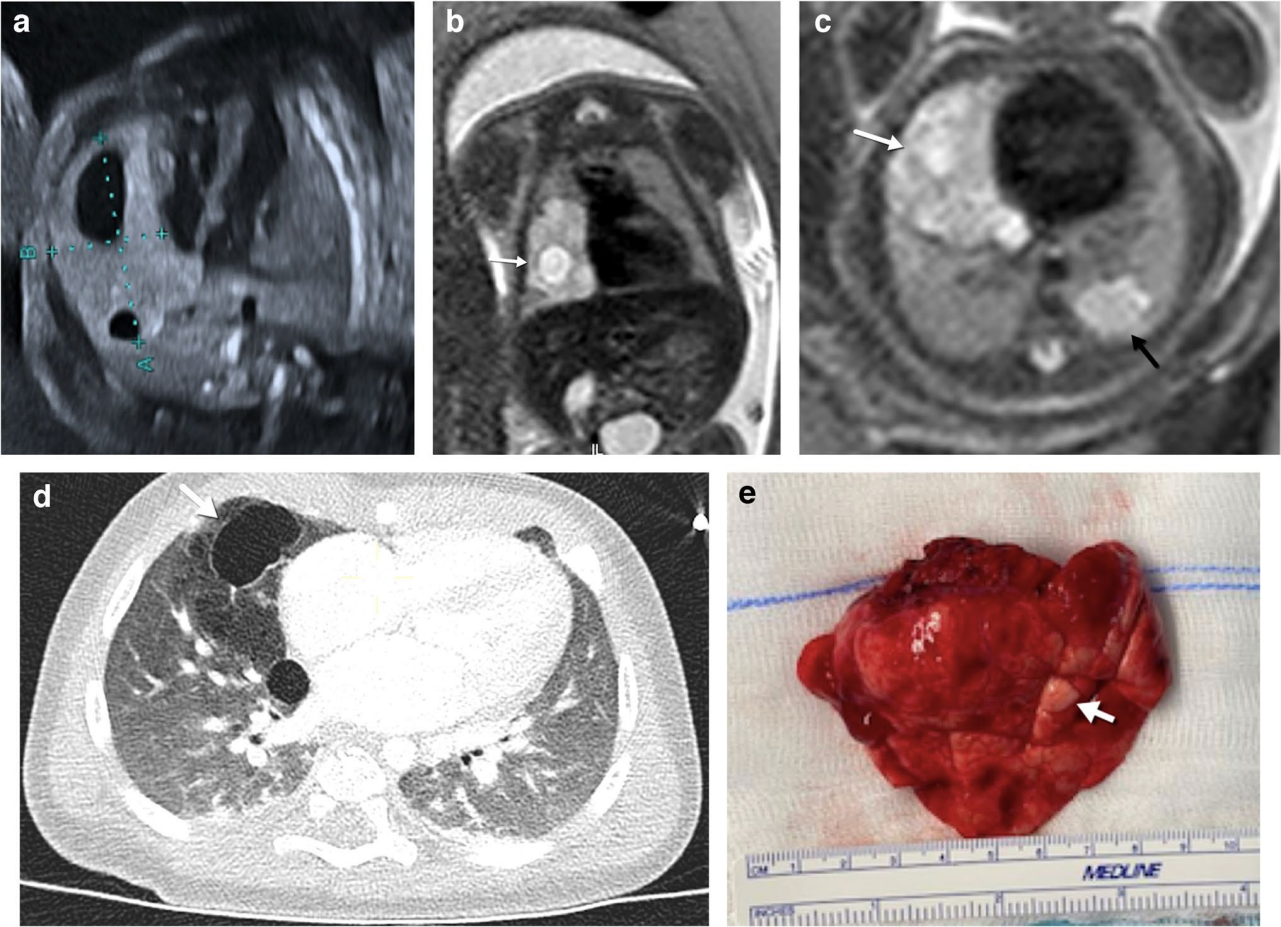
A congenital pulmonary airway malformation in a 22-week gestational age male fetus. a Axial ultrasound image of the chest shows a hyperechoic lung lesion with two macrocystic lesions in the middle lobe of the right lung. b, c Fetal magnetic resonance imaging was performed the same week. Single-shot T2 coronal (b) and axial (c) images confirm the presence of a lung mass with multiple cysts in the middle lobe and a second lesion in the left lower lobe. The lesion located in the middle lobe has a dominant macrocyst (*white arrows*), whereas the lesion in the left lower lobe contains microcysts (*black arrow in *c). No signs of hydrops or mediastinal shift are noted. These lesions were treated with intramuscular injections of steroids to the mother. d An axial postnatal computed tomography image (lung windows) obtained at 6 months of age confirms the presence of a cystic lung lesion in the middle lobe. f Surgical photograph after middle lobectomy demonstrates congenital pulmonary airway malformation type 1 with multiple cysts (*arrow*)
